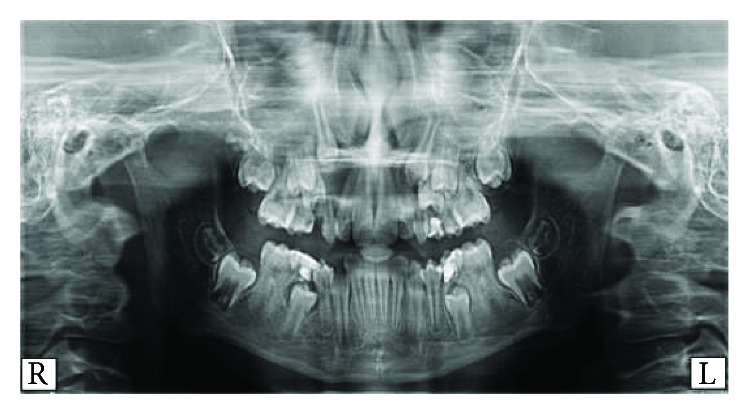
Older orthopantomogram (OPG) showing the absence of any radiolucent lesion at the mandibular left side (second premolar region).
